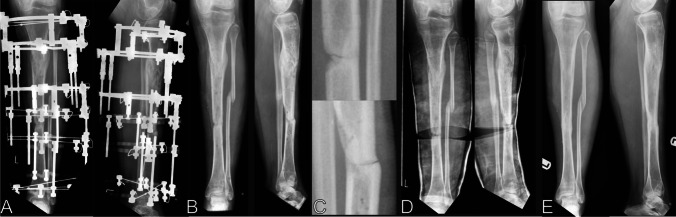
Docking site refracture managed non-operatively. A Patient has apparently successfully completed treatment for a Gustilo and Anderson IIIB open tibial fracture with bone loss. Note consolidated proximal transport segment and mid-diaphyseal docking site which appears united after simple compression. B Radiographs early after frame removal appear to show a united tibia. C Patient stumbles and suffers a low energy fracture. Radiographs reveal a minimally displaced refracture at the docking site. D A plaster cast is applied and wedged to restore alignment. E Spontaneous union of the refracture occurs over 4 months. Note that the docking site has better union than prior to the refracture
